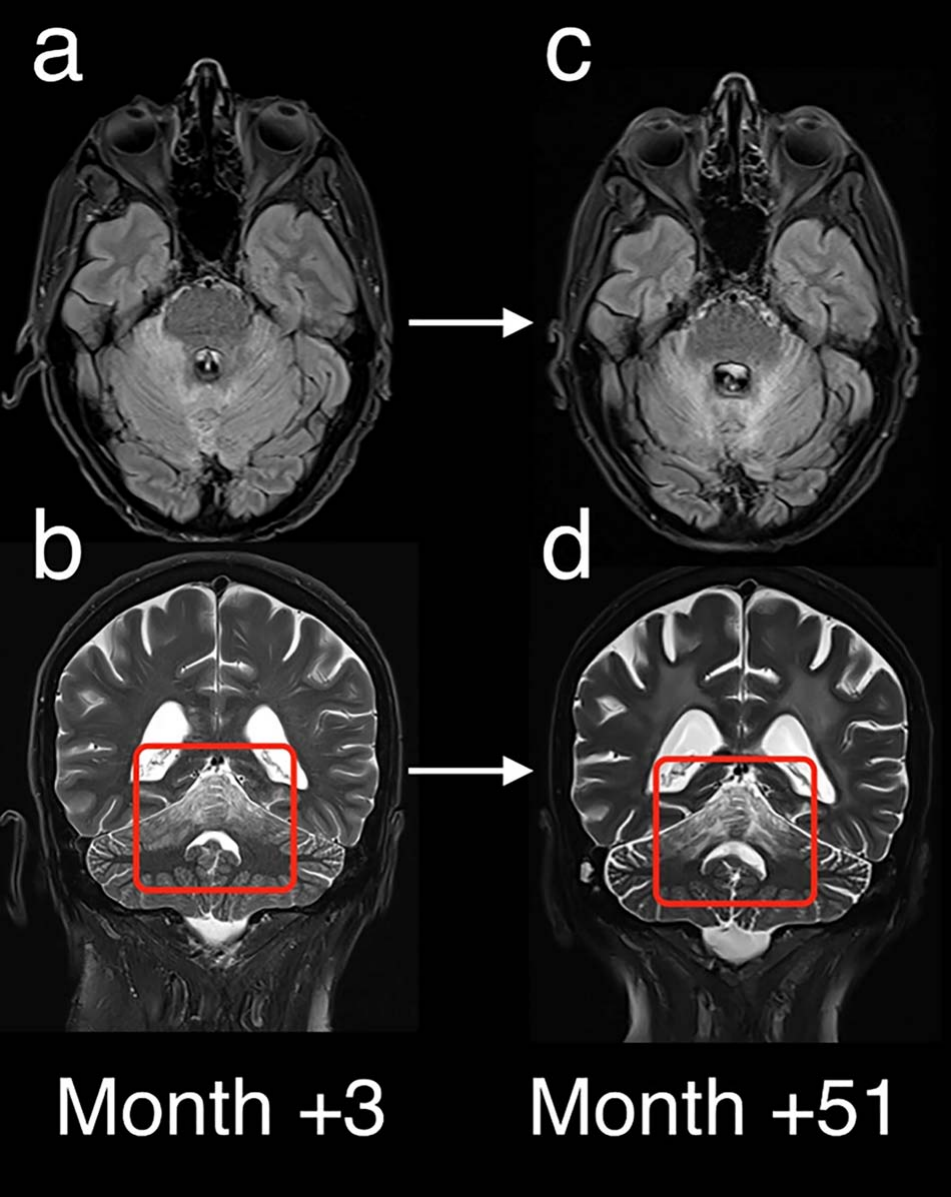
Long-term follow-up imaging with Axial T2 FLAIR (a/c) and Coronal T2 (b/d) shows stable T2 FLAIR signal and stable T2 signal with mild interval atrophy of the vermis (red box). No evidence of recurrence or progression at 51 months after chemoradiotherapy and adjuvant Sonidegib.
